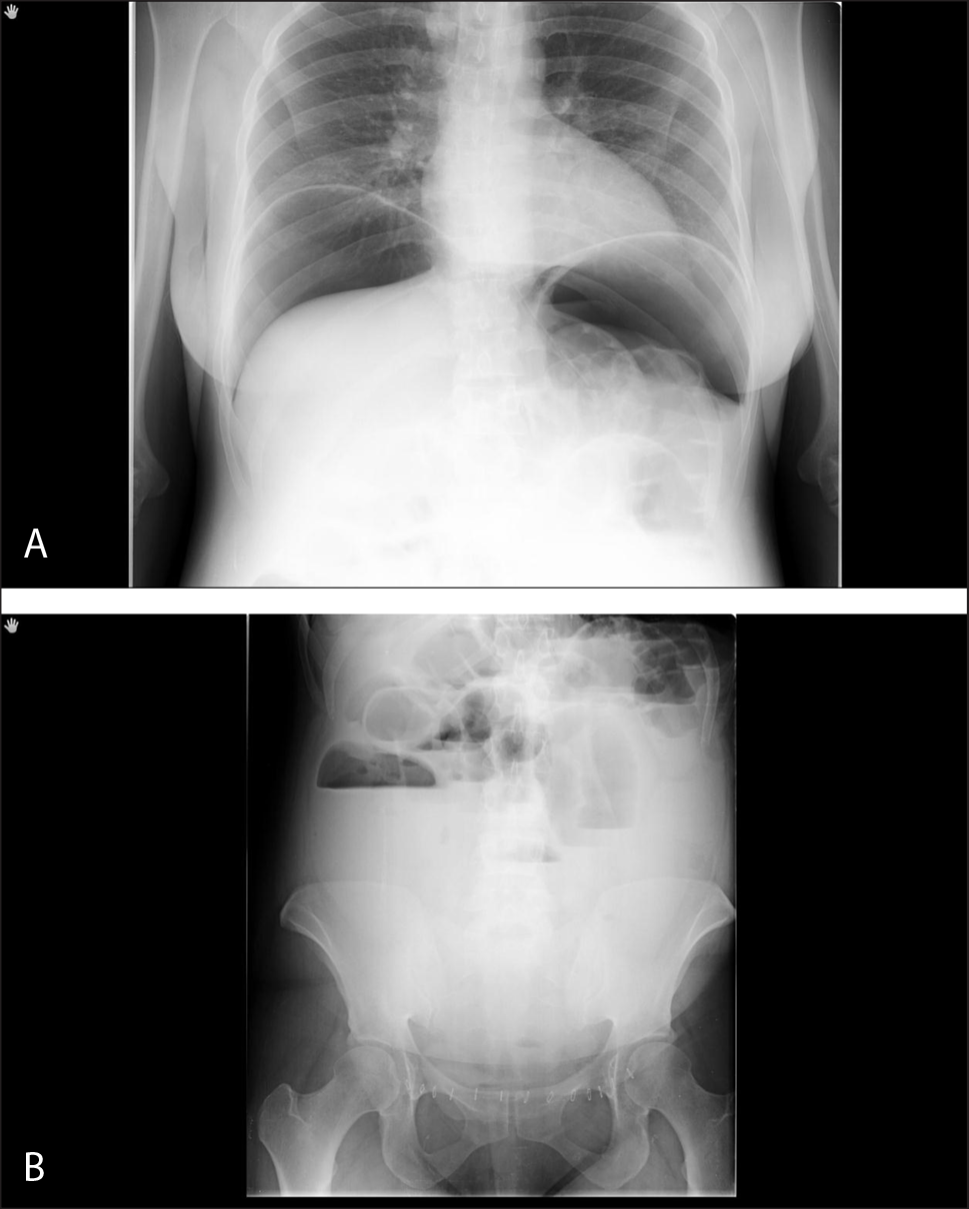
Plain abdominal X-ray showing an important pneumoperitoneum and hydroaeric levels in the intestines.
